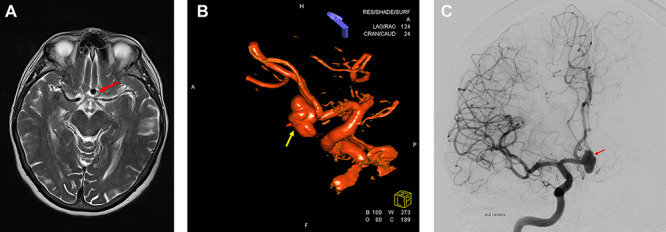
Preoperative neuroimaging. (A) MRI T2-weighted sequence shows an Acom aneurysm without thrombosis. (B, C) Digital subtraction angiography shows a multi-lobulated Acom aneurysm approximately sized 13.0 × 7.5 mm. (B) 3D Digital subtraction.
